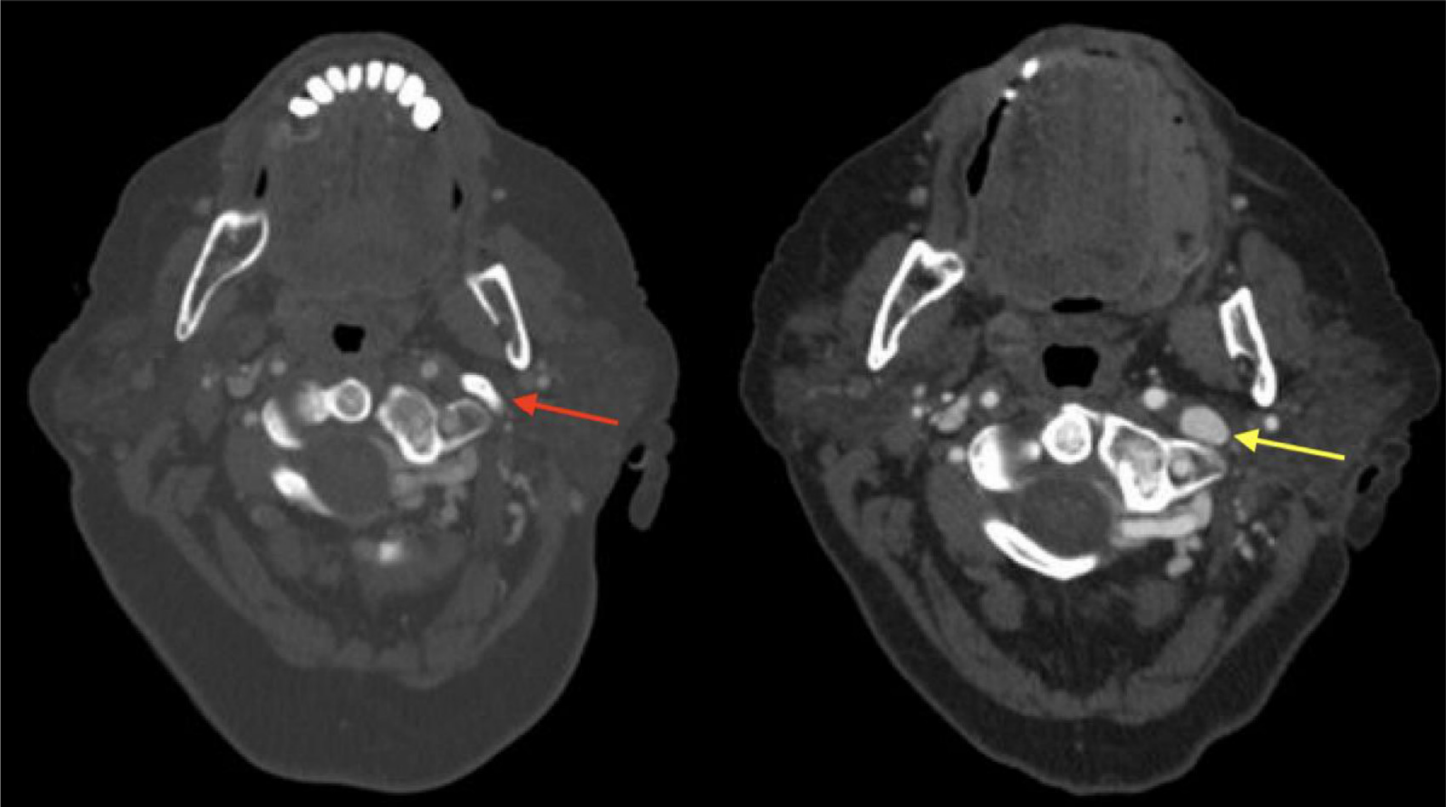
(A) (right): Preoperative CTA demonstrates calcified stylohyoid ligament with absence of the left internal jugular vein. (B) (left): yellow arrow demonstrating decompression of L IJV after styloidectomy.
